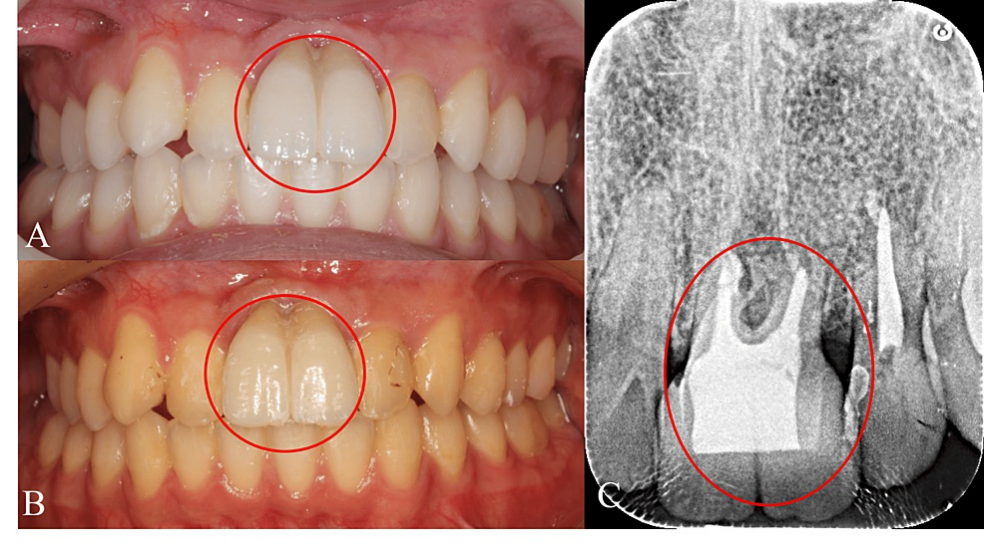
Intraoral photograph after setting a final prosthodontic restoration (A) Immediately after setting a final prosthodontic restoration. (B) The intraoral photograph taken four years after autotransplantation. (C) Dental X-ray taken four years after autotransplantation. The encircled region shows the autotransplanted tooth.
